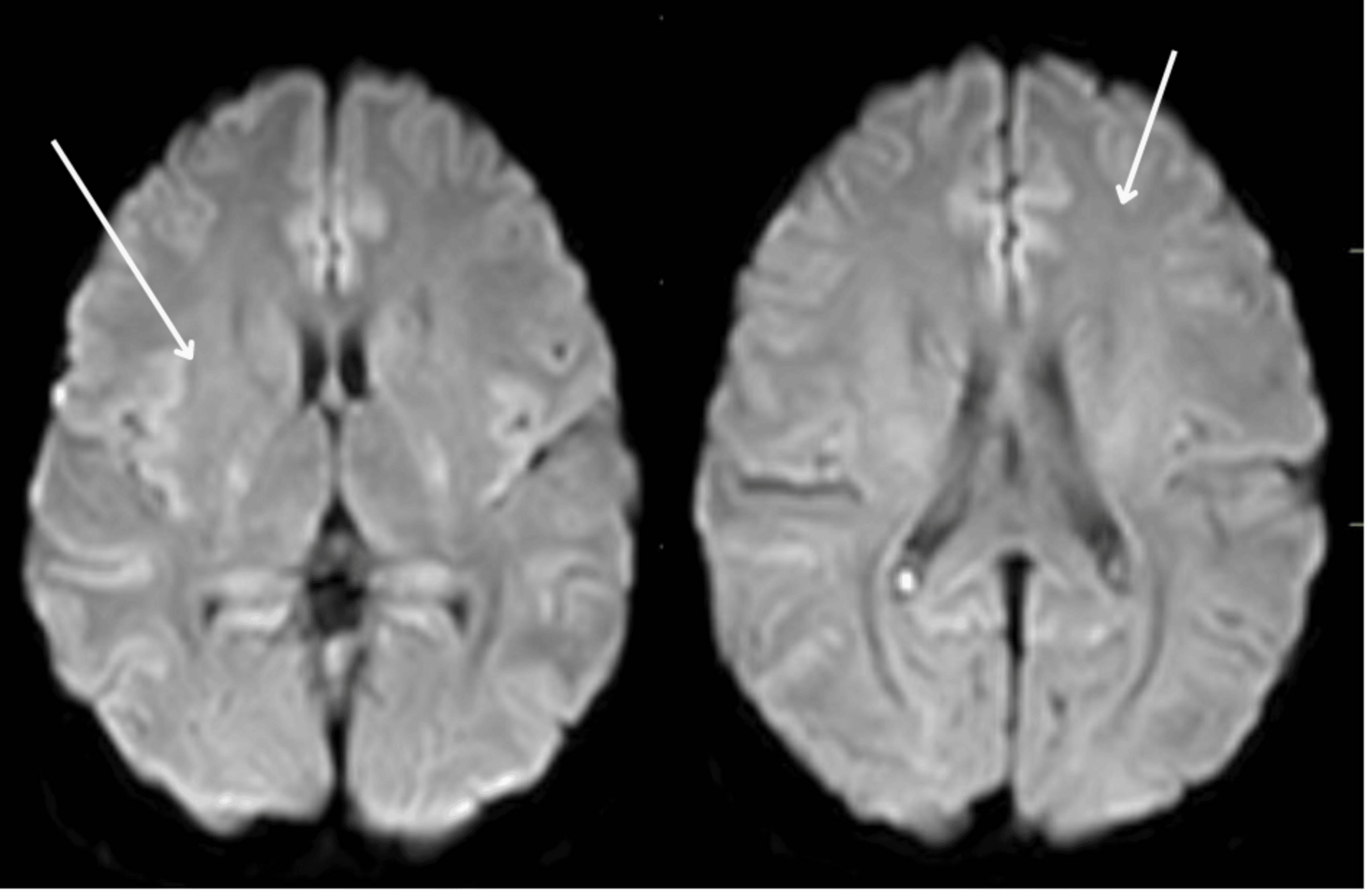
Magnetic resonance image of brain (diffusion-weighted image) with normal grey white differentiation in the brain parenchyma indicating the resolved cerebral edema (white arrows)
